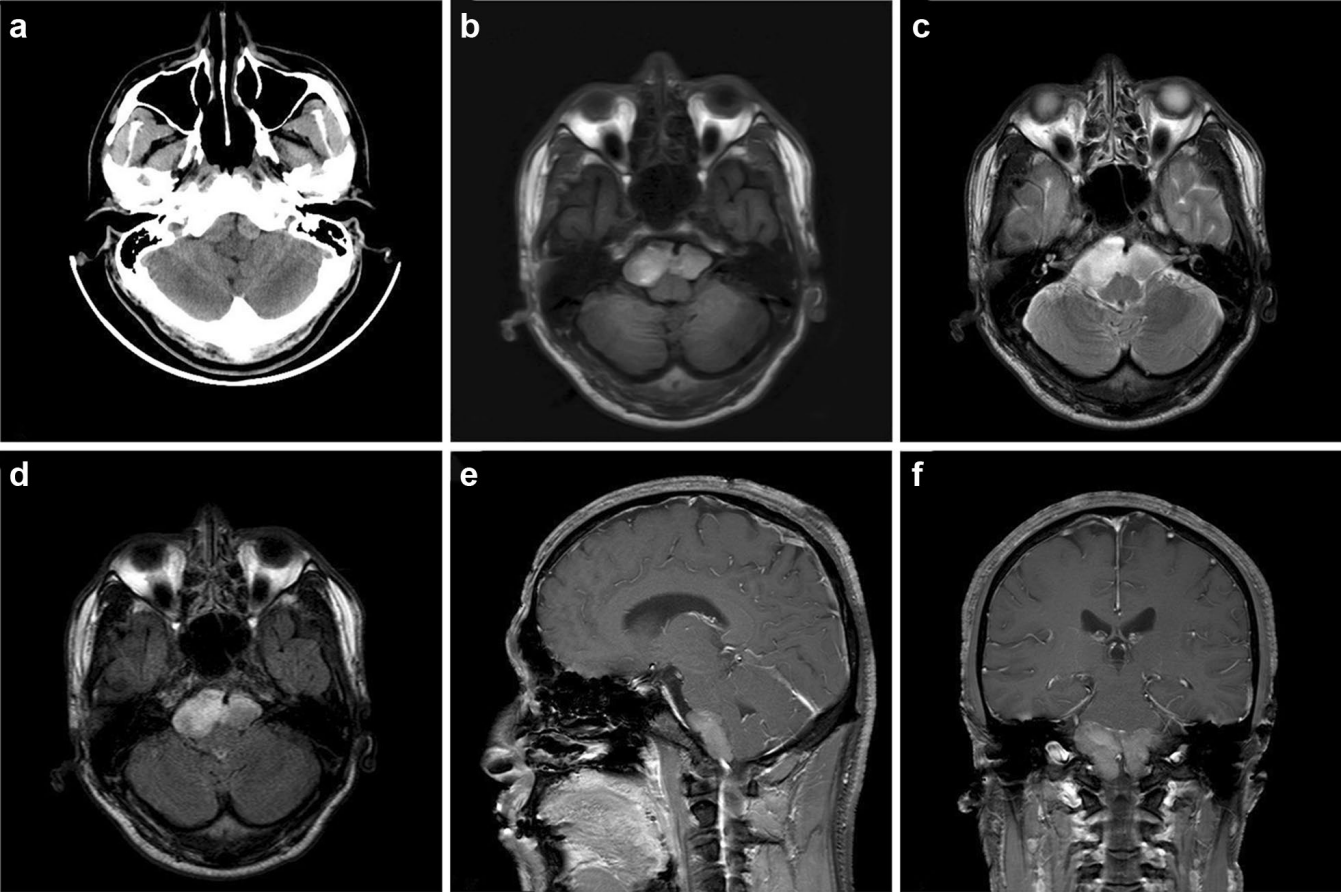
Preoperative computed tomography and magnetic resonance imaging: a axial non-contrast computed tomography (CT) scan showing a slight hyperdense lesion lying in the prepontine cistern; b, c axial T1- and T2-weighted magnetic resonance imaging (MRI) demonstrated a hyperintense mass in the prepontine cistern, the basal artery was enveloped and the brainstem was compressed; d axial FLAIR image shows a hyperintense extra-axial cyst compared with CSF; e, f sagittal and coronal enhanced MRI scans demonstrate a heterogeneously non-enhanced ovoid mass located ventral to pons and medulla
